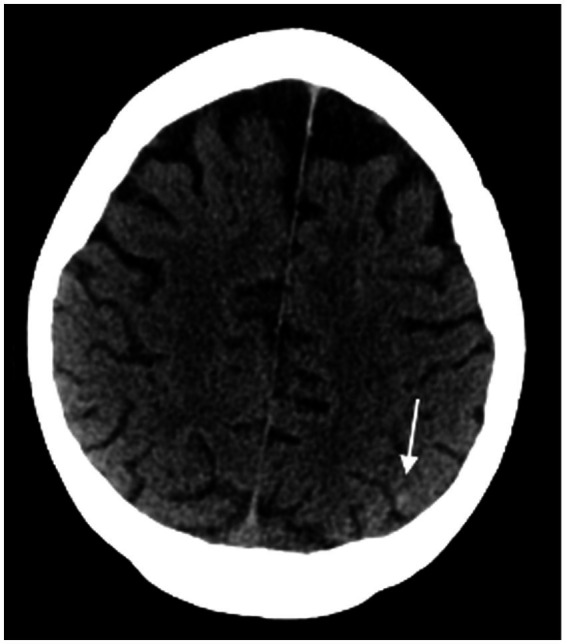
Computed tomography axial section of the head demonstrates a subtle punctate hyperdense focus in the left parietal cortex without any other observable lesions, suggesting a punctate intracerebral hemorrhage.
